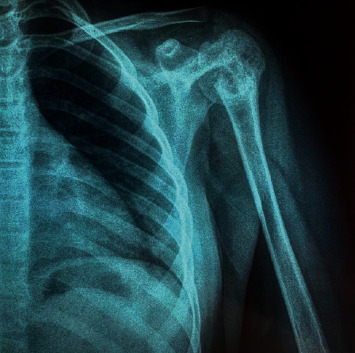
Plain film reveals osteolytic metastases in the left humerus.
